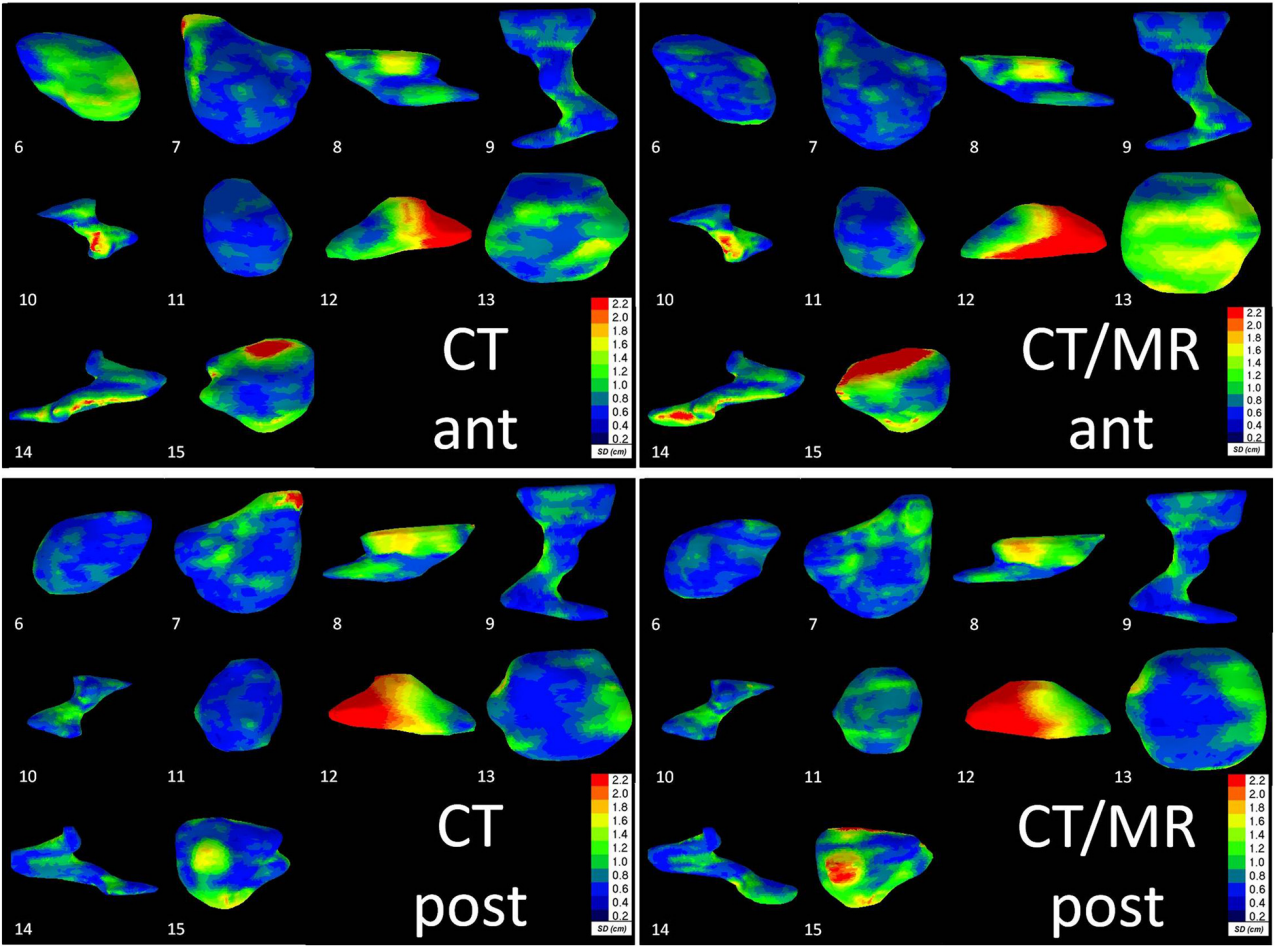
Left: Coronal view of the ten delineated Lumpectomy Cavity (LC) CT volumes in both anterior as well as posterior view. Right: Coronal view of the ten delineated LC CTMR volumes in both anterior as well as posterior view. The local surface distance variation of the four observers is projected on the median surface of each LC. Colour map: Blue: high agreement between observers; Red: low agreement between observers according to the scale given.
